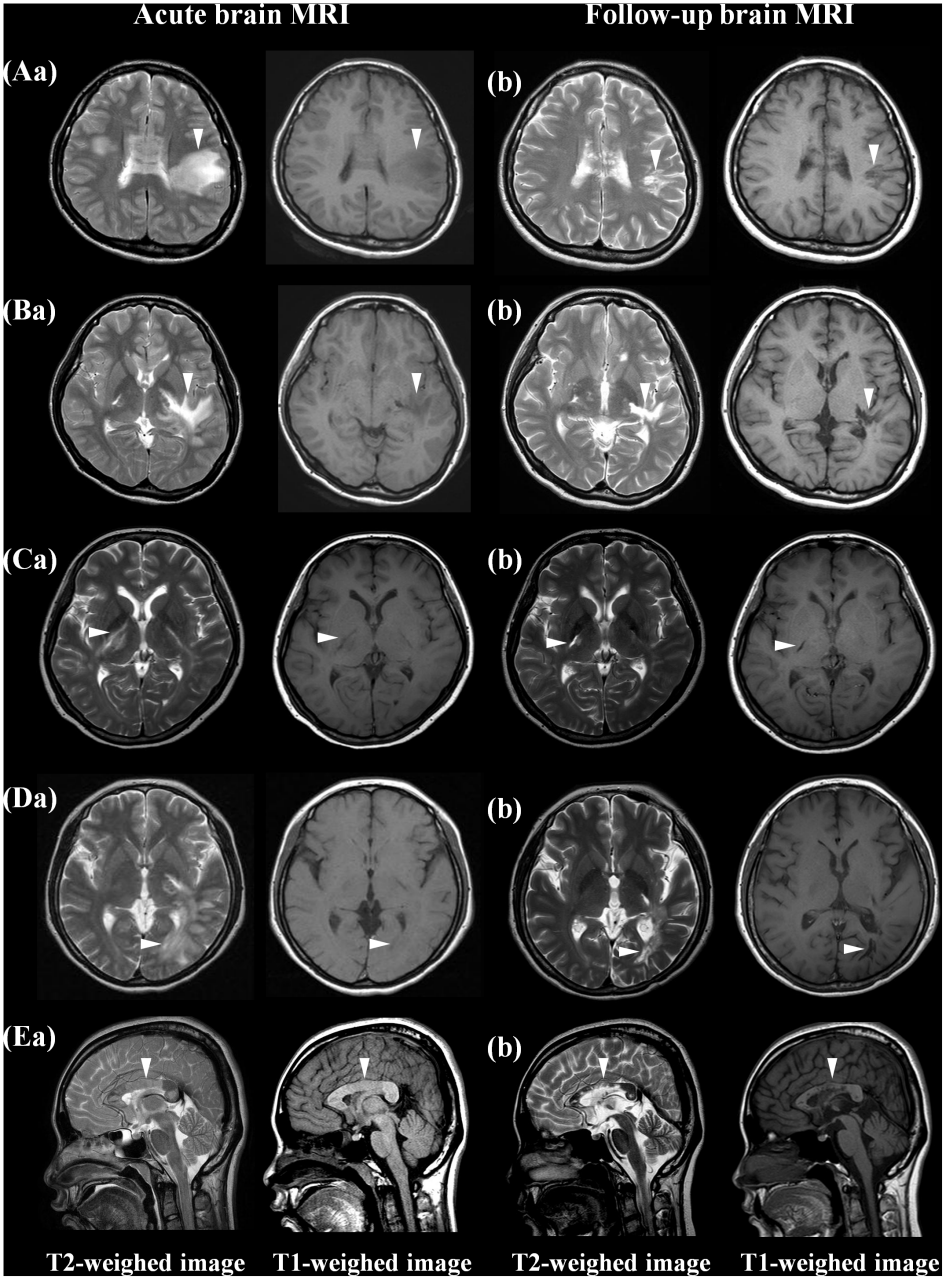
Chronic cystic changes of brain lesions on MRI. At the time of an acute brain attack, brain MRI showed multiple T2-hyperintense lesions with subtle T1 hypointensity in the frontal white matter (Aa), corticospinal tract (Ba, Ca), occipital white matter (Da) and corpus callosum (Ea). On follow-up MRI, all T2 hyperintense lesions were markedly decreased in size but revealed focal T1-hypointensity with cystic changes (Ab, Bb, Cb, Db, Eb).
